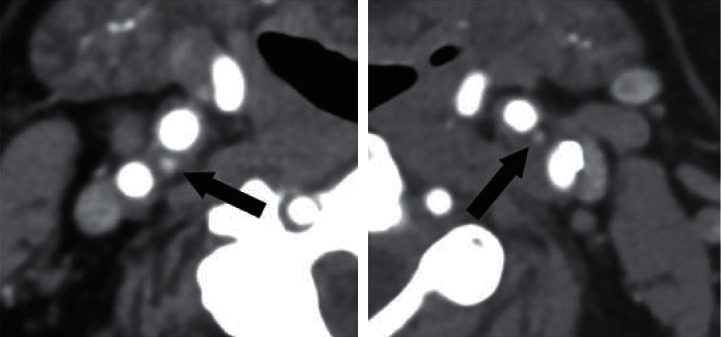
CTA shows moderately enlarged carotid bodies (arrow) on both sides in a 54-year-old woman who suffers from high blood pressure.
